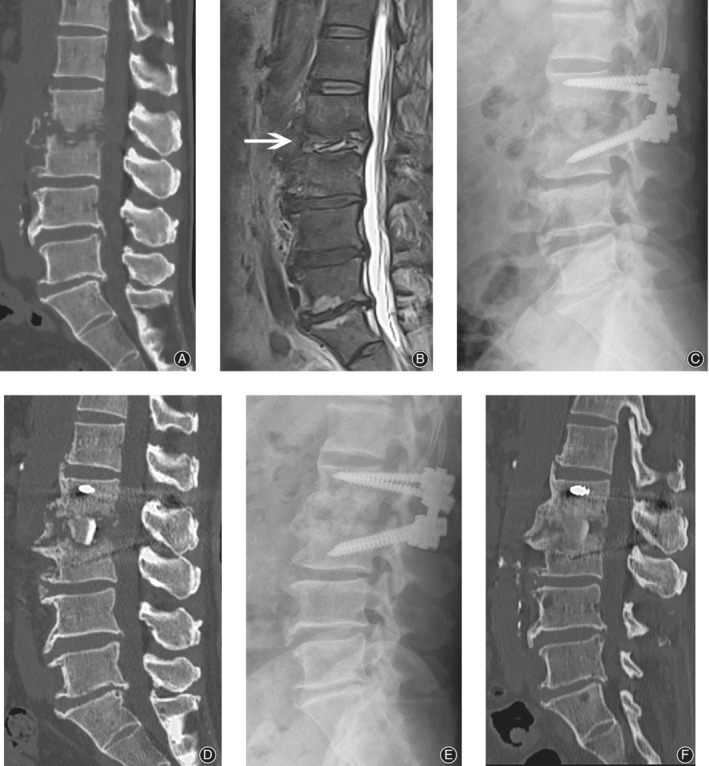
Sixty‐two‐year‐old woman, whose chief complaint was back pain of more than 3 months. Pre‐operative CT (A) and MRI (B) images revealed L_2–_
